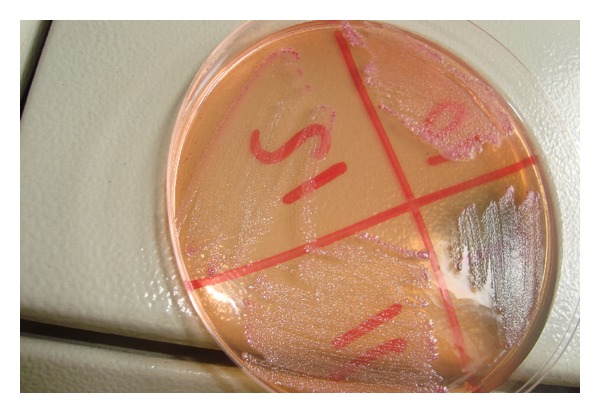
Sorbitol-nonfermented colonies of *E. coli *O157:H7 on CT-SMAC media.
